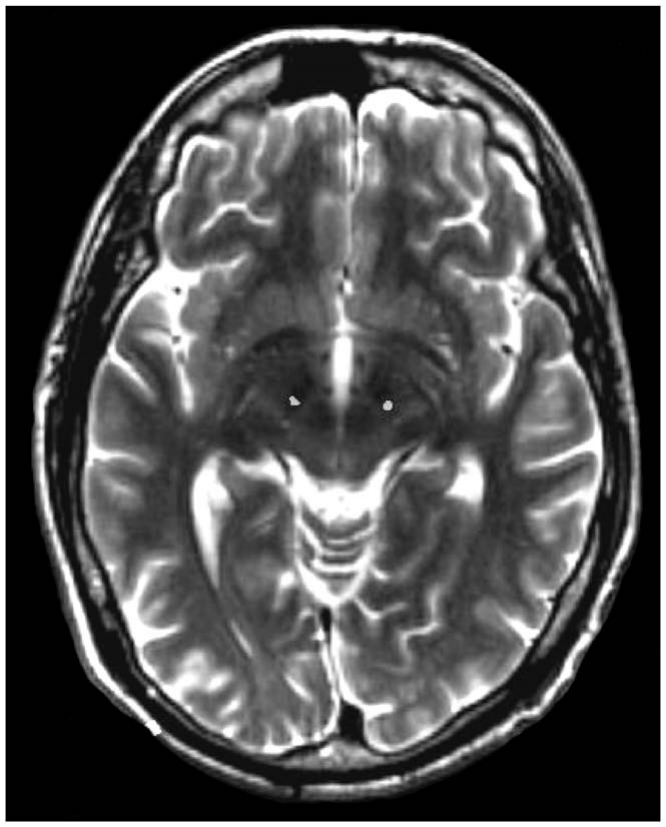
Confirmation of lead position: Postoperative thin slice CT windowed to show only the radio-dense leads (bright white spots) and fused to the preoperative T2 weighted MRI scan.
